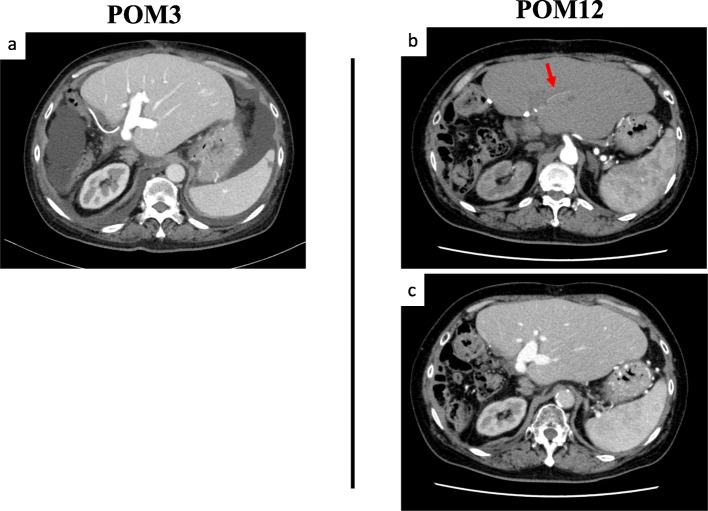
a CT scan performed on POM 3 showing that the portal vein was enhanced through the ileal arteriovenous shunt at the arterial phase. b CT scan performed on POM 12 showing that a thin hepatic artery (arrow), which indicated improved arterialization through bile duct anastomosis, was visible during the early arterial contrast phase. c The portal vein was enhanced through the ileal arteriovenous shunt on the late arterial phase
